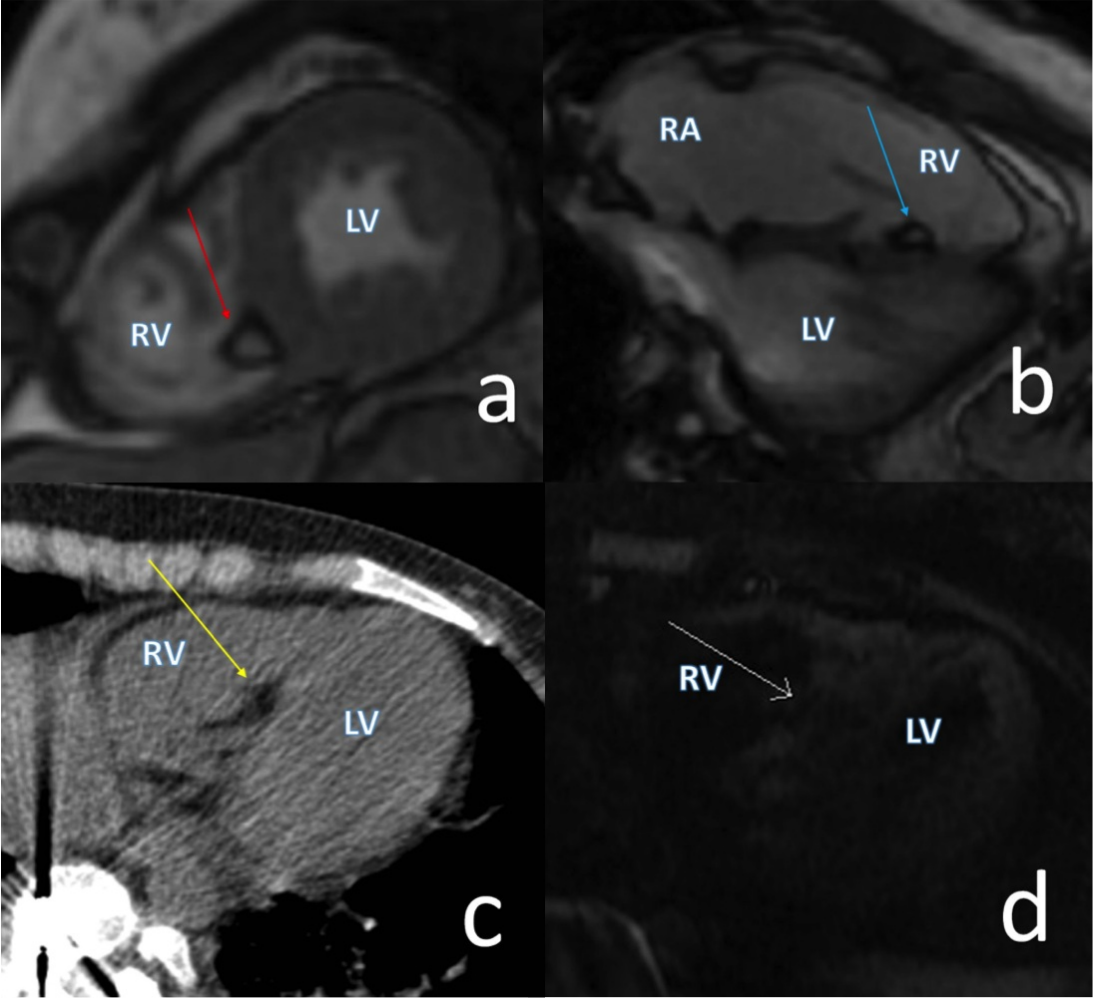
Cardiac CT and MR of an Intracardiac Lipoma (a) A bSSFP cardiac MR image in a two-chamber short axis view demonstrates a subendocardial focal fatty deposit in the midportion of the interventricular septum with associated chemical shift artifact (red arrow). (b) Similar finding demonstrated on the bSSFP four-chamber horizontal long axis view (blue arrow) and (c) Unenhanced axial CT (yellow arrow). (d) Axial TIR (fat saturated) image demonstrates loss of signal in the subendocardial deposit (white arrow). bSSFP = balanced stready-state free precession; TIR = triple inversion recovery; RA = right atrium; RV = right ventricle; LV = left ventricle.
